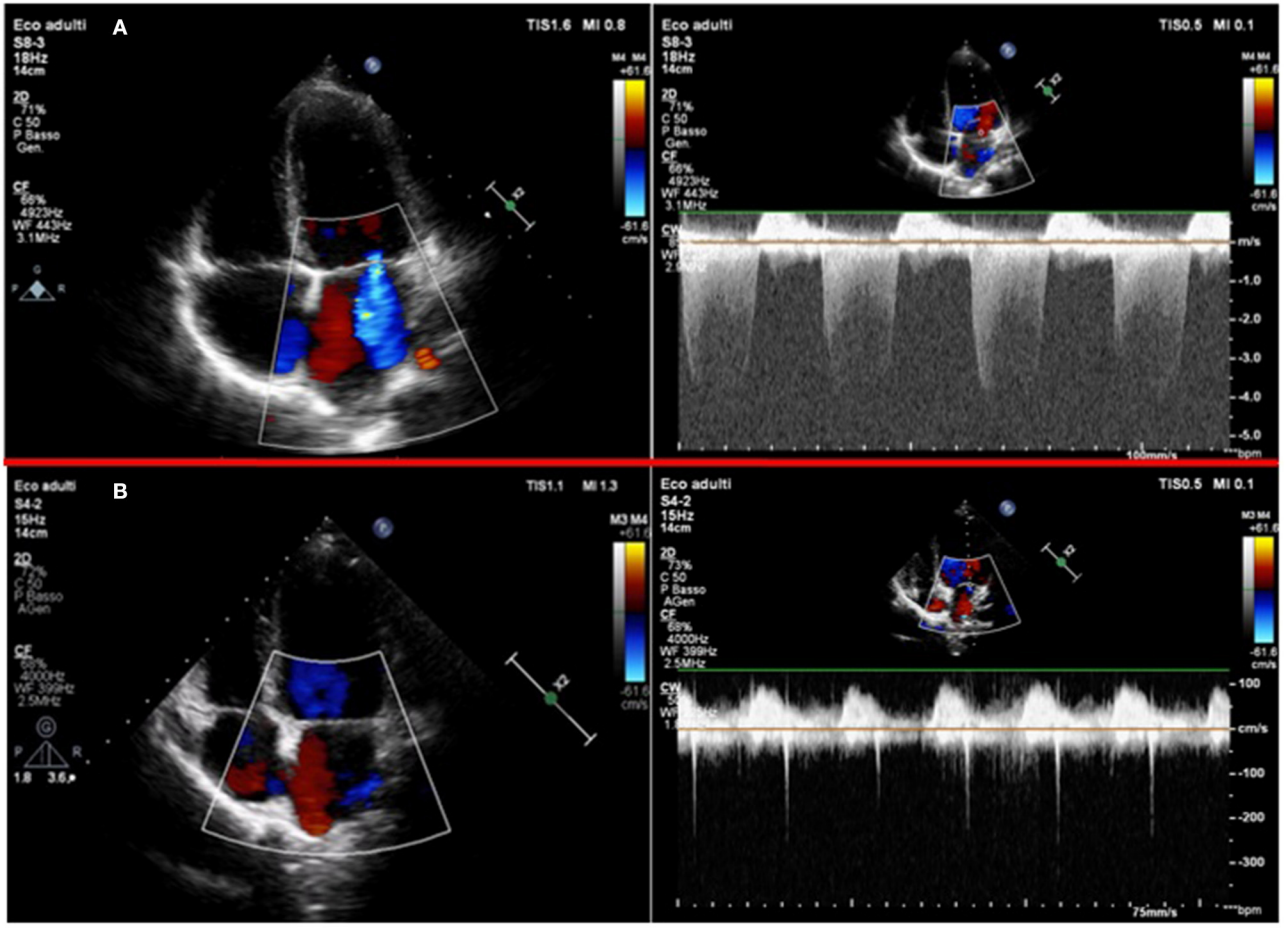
(A) 2-D Color-Doppler echocardiography of the first patient in the acute phase: left panel shows the apical 4 chambers view with color flow Doppler, showing a moderate mitral regurgitation; right panel shows the continuous wave Doppler of the mitral regurgitant jet. (B) 2-D Color-Doppler echocardiography of the same patient 1 month after: left panel shows an apical 4 chambers view with color flow Doppler and right panel shows the continuous wave Doppler of the minimal mitral regurgitation.
